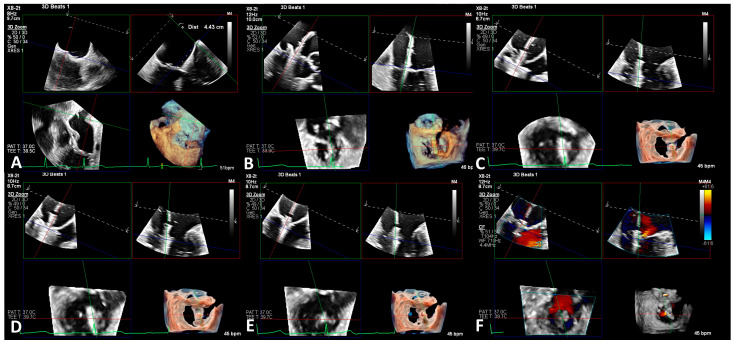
(A–E) shows the critical steps in mitral transcatheter edge-to-edge repair. A desirable transeptal puncture (TSP) tends to be at the posterior and superior to mid aspect of the fossa ovalis, though this is highly dependent on interatrial septal anatomy, the SVC/IVC trajectory and the relationship of the fossa ovalis to the mitral valve. The TSP shown in (A) is at the mid/superior portion of the fossa ovalis. A height of >4.0 cm above the medial commissure of the mitral valve is typically necessary. (B) shows the clip trajectory, as well as orientation prior to bringing the clip to the ventricular side of the mitral valve. (C) shows clip orientation as well as the grasping view in the green box (upper left, within (C)). In (D), the anterior and posterior mitral leaflets were captured with the clip arms, and the clip was subsequently closed (E). (F) is a 3D color Doppler interrogation of the mitral valve after deployment of one clip with trace residual MR.
